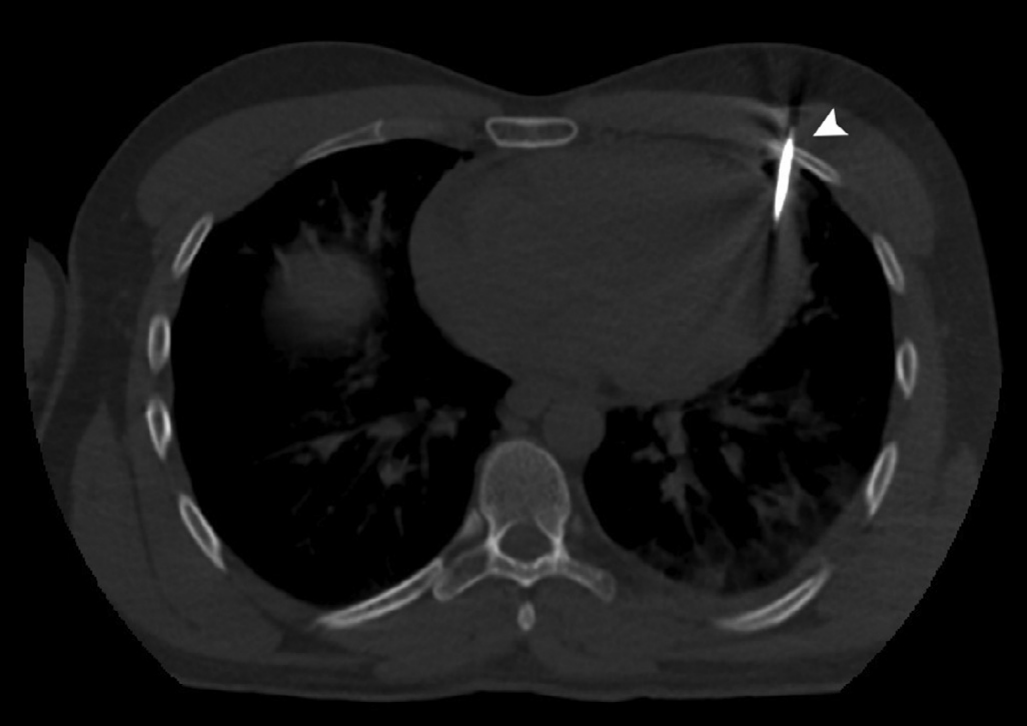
Mediastinal window of the chest CT demonstrated left ventricle wall and cavity penetration by nail (white arrowhead).
